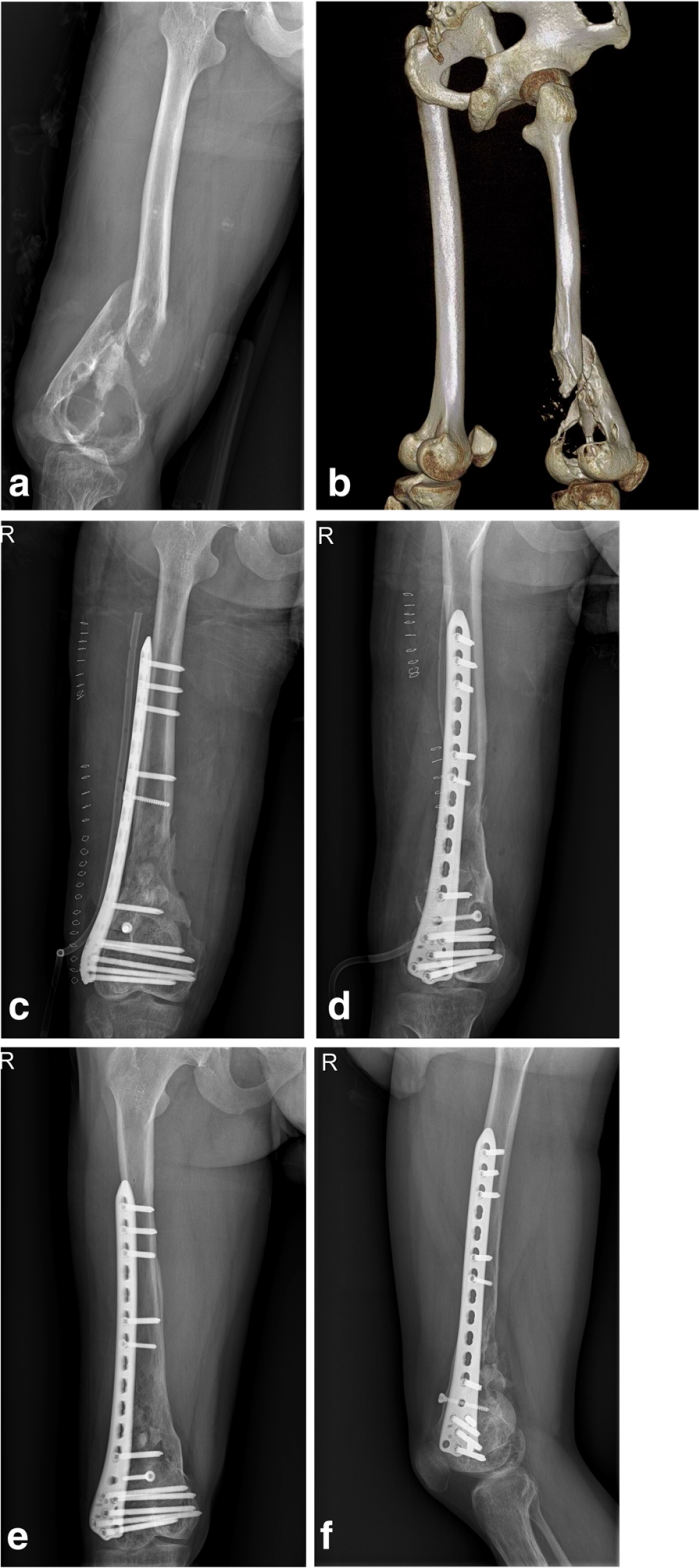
Surgical treatment with locking plate and allogeneic cortical strut graft was applied to a 23-year-old man (case 4) with a right distal femoral HPT-related fracture. Radiographs were taken at the a, b preoperative, c, d postoperative, and e, f 20-month postoperative timepoints
